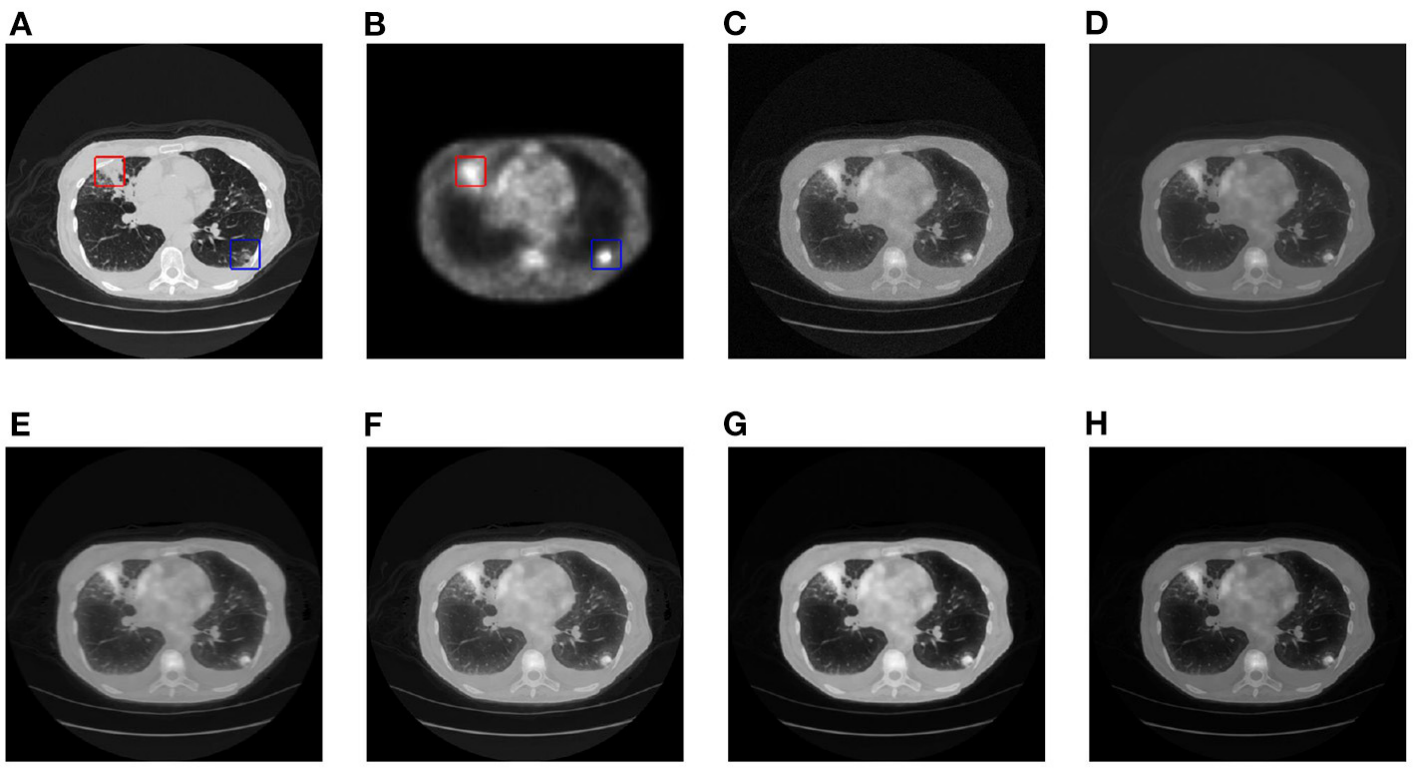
The qualitative comparison results of patient A. (A) CT; (B) PET; (C) AD; (D) GF; (E) PAPCNN; (F) MCFNET; (G) IFCNN; (H) OURS.
